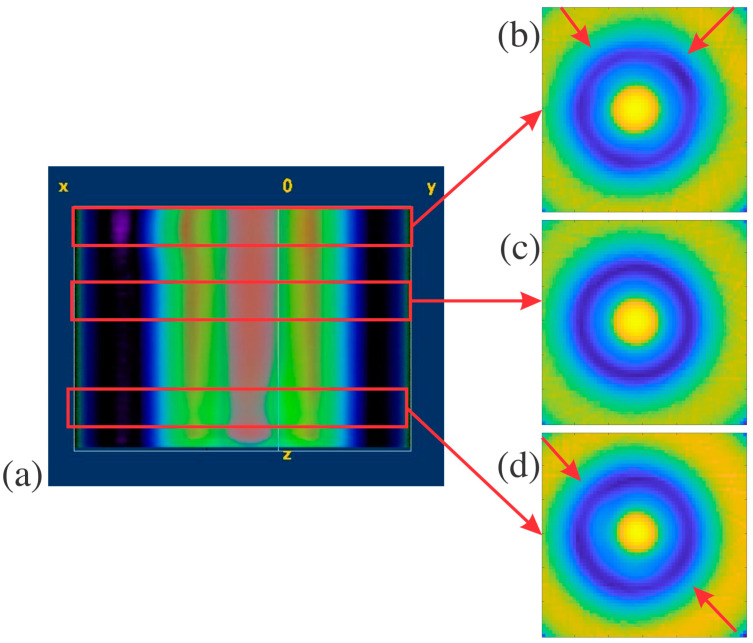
Structure B THz CT slice reconstructions in FIJI and MATLAB: (a) shows a FIJI reconstruction of structure B with internal and external defects, used to identify the location of the defects, with red arrows pointing to different cross-sectional areas of the reconstruction, (b) MATLAB plot of structure B with an external defect and inconsistencies pointed out by the red arrows, (c) MATLAB plot of a structure B slice without defects, and (d) MATLAB plot of a structure B with an internal defect and inconsistencies pointed out by the red arrows.
